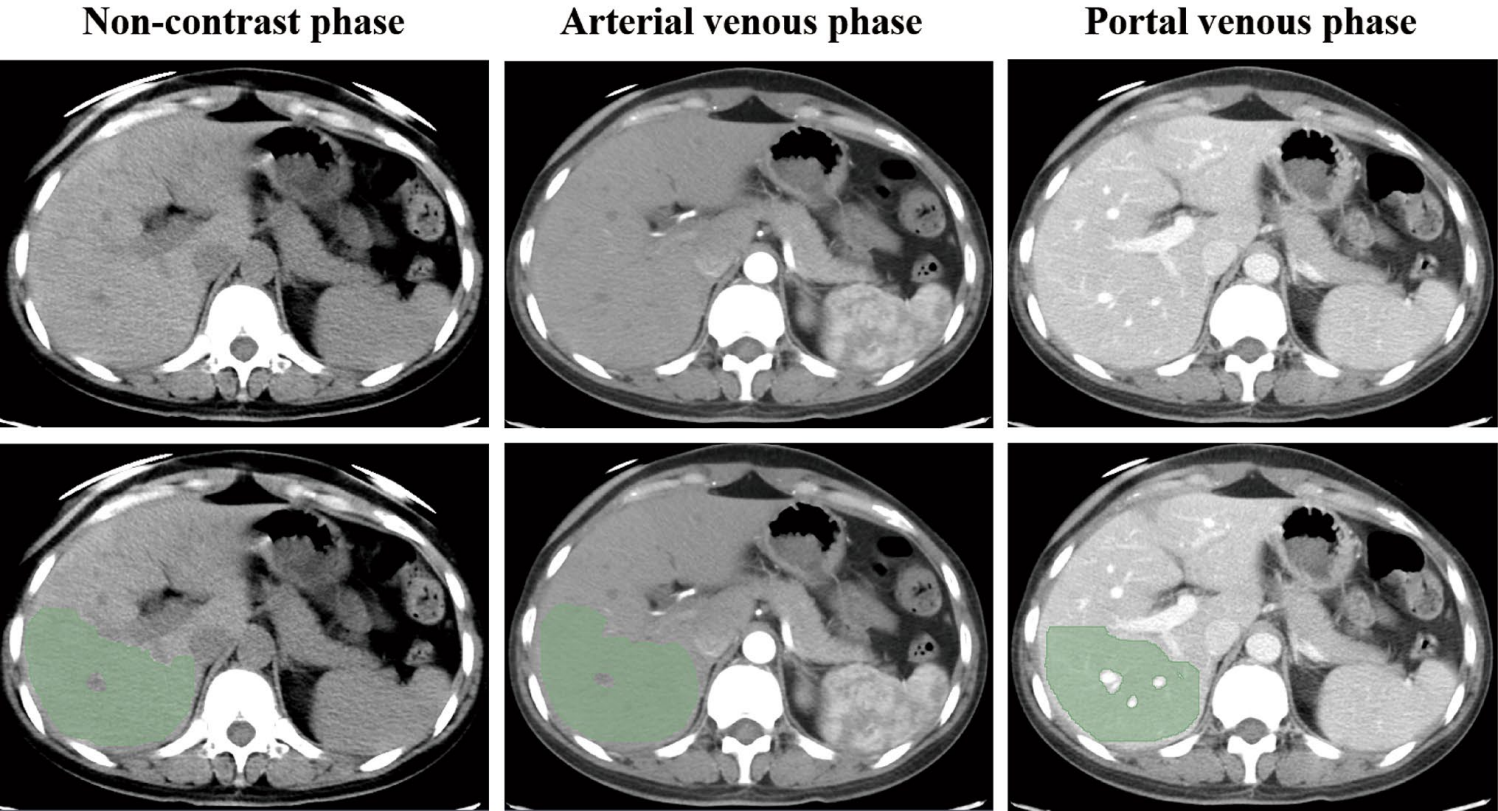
ROIs for the liver at contrast-enhanced CT. ROIs were delineated along the margin of the right hepatic lobe, at the level of the right portal vein, by excluding large hepatic vessels and masses on non-contrast, arterial and portal venous phases CT images. *CT* computed tomography, *ROI* region of interest
